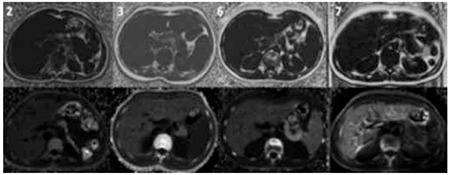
The magnetic resonance imaging-proton density fat fraction (top row) and R2* (bottom row) maps of the patients with milder hepatic iron overload. There is no artifact in patients with milder hepatic iron overload.
